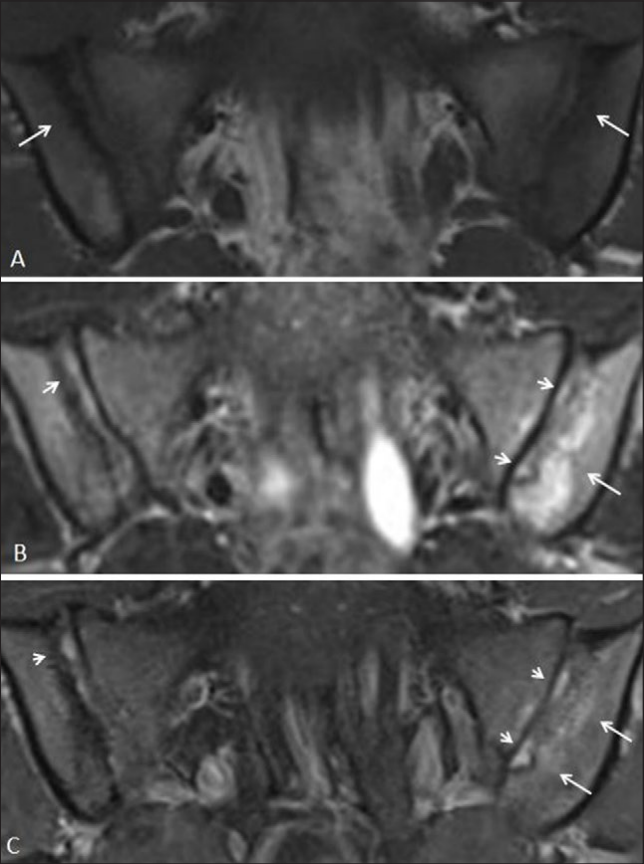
Active and structural sacroiliitis in a 15-year-old boy with JSpA. a. Semicoronal T1-weighted image shows erosions on both SI joints and subchondral sclerosis at the iliac side of the right SI joint (arrows). b. Semicoronal STIR image shows high signal in the joint space of both SI joints (short arrows) and bone marrow edema at the left iliac side (arrow). c. Contrast-enhanced fat-saturated T1-weighted image shows synovial enhancement in the erosions (short arrows) and enhancing osteitis (arrows).
